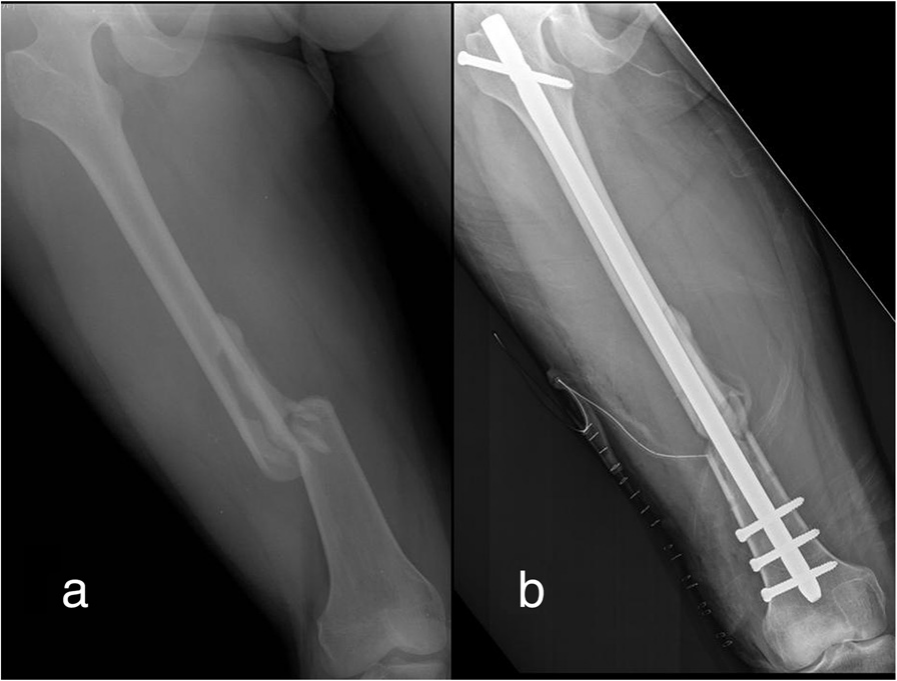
(a) Radiograph of the femur showing a comminuted, spiral fracture over the shaft before operation; and (b) a satisfactory fixation was obtained after a lengthy closed femoral nailing
